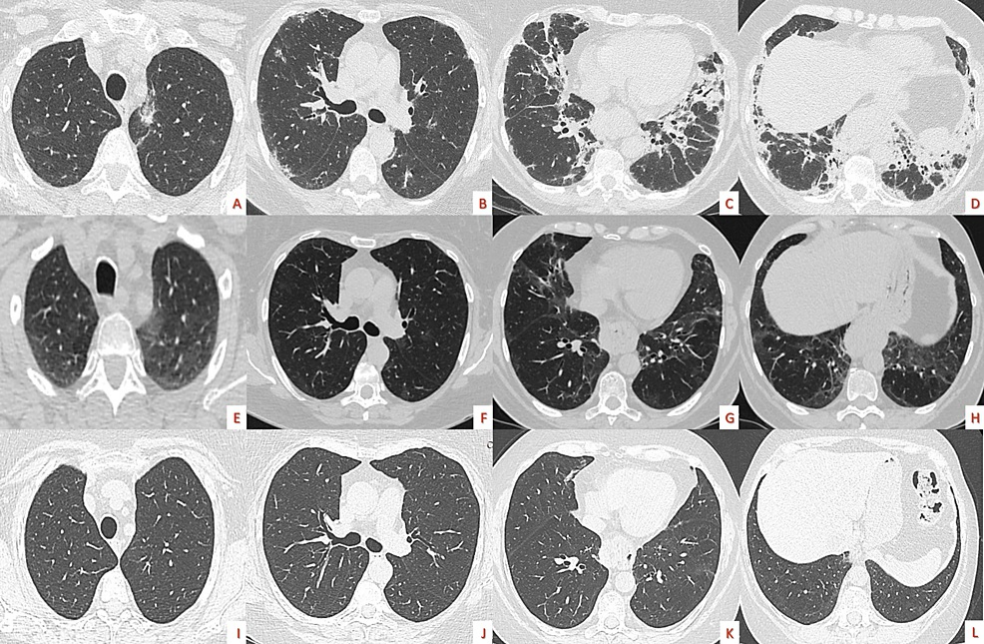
Serial follow-up high-resolution computed tomographies after therapy completion Axial slices from lung apices to bases of chest computed tomographies showing a radiological improvement; steroid naive (A-D), after one month of therapy (E-H), after four months of therapy (I-L).
